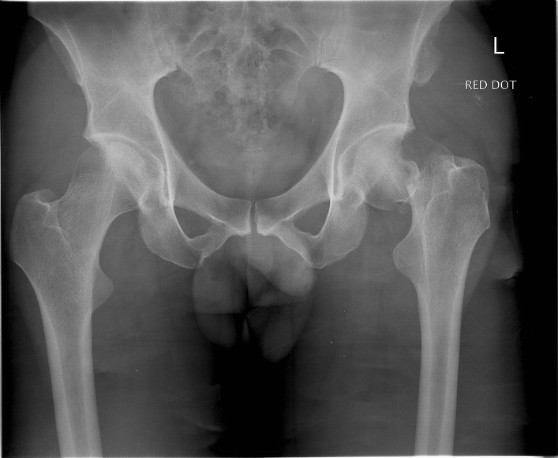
Pelvic radiograph view showing fracture to his left proximal femur.
